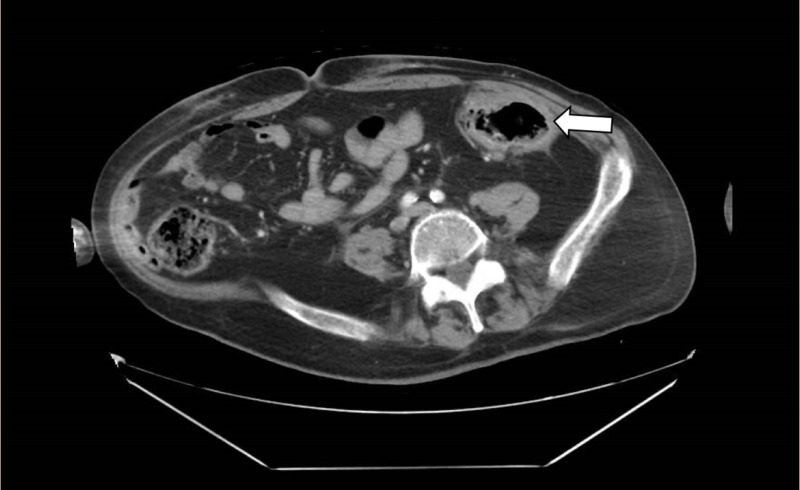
Axial view of an enhanced abdominal CT scan showing a large thin-walled cyst with air-fluid levels arising from the sigmoid colon (arrow)
